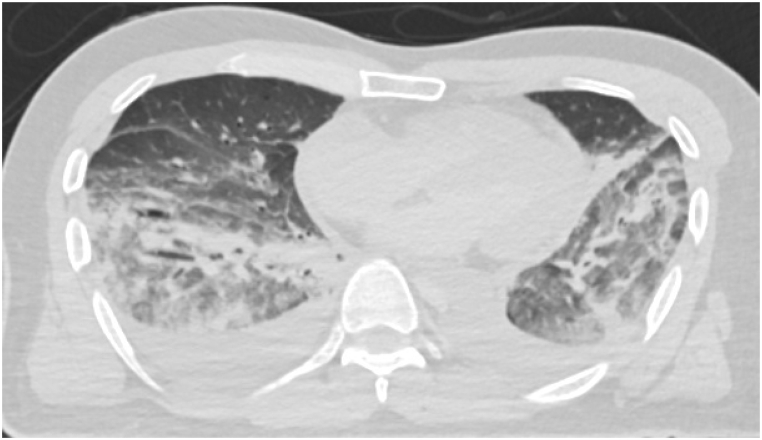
Postmortem computed tomography images. Axial image of the chest. Dorsal pulmonary infiltrative shadow and bilateral pleural effusions were detected. Cardiothoracic ratio was 55 %.
